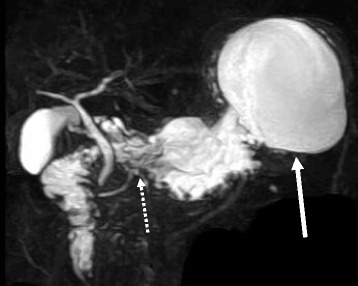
MRCP revealed a simple cyst of the pancreatic tail like CT images (*arrow*). No pancreatic tumor could be detected around the stenosis of MPD. The distal MPD was not clearly visualized (*arrow*)
